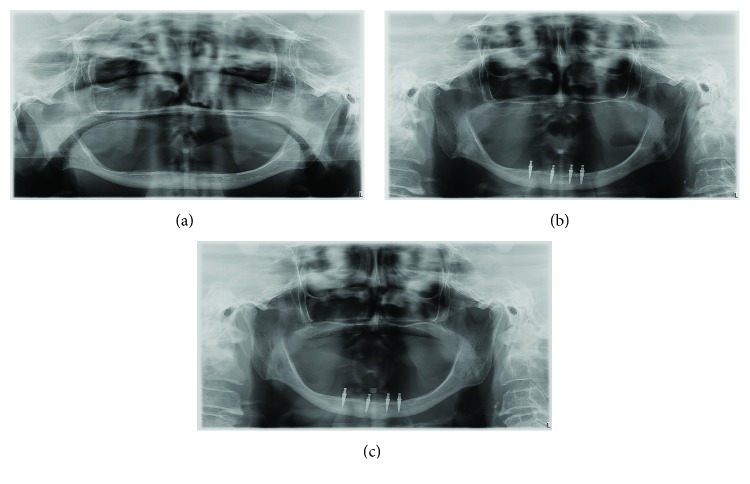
Panoramic radiographs (a) before treatment, (b) after one year, and (c) after 2 years of wearing the mandibular overdenture.
